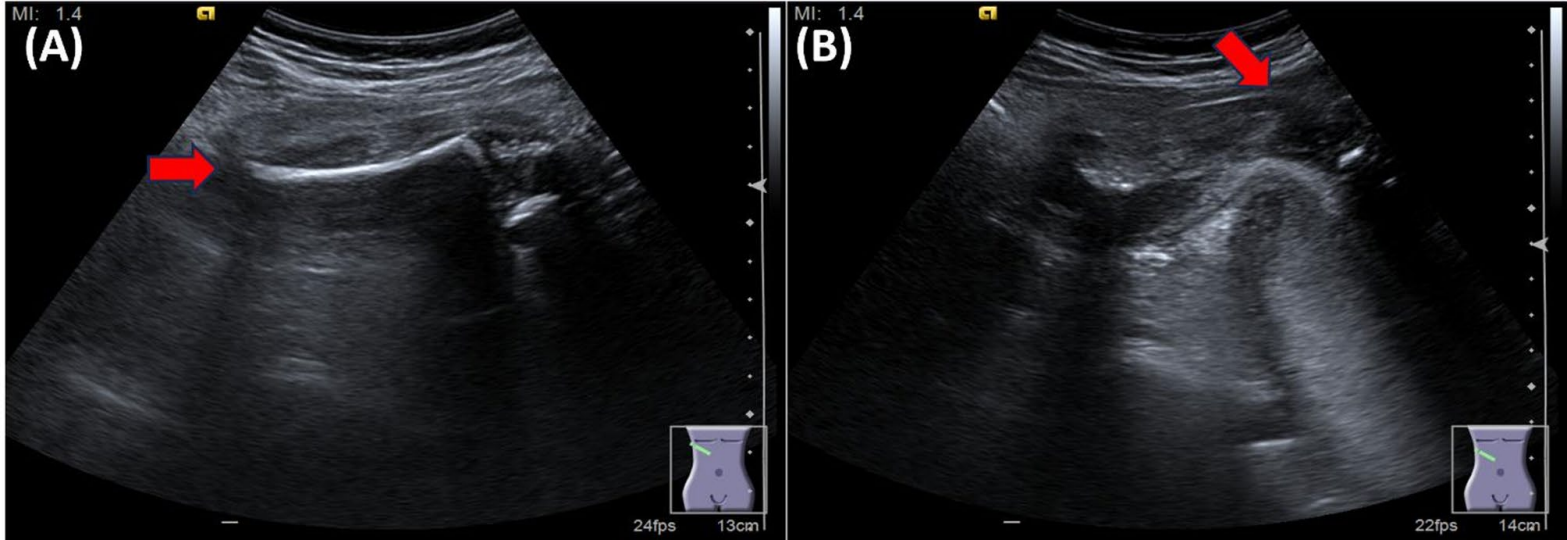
Ultrasonographic findings suggestive of uterine rupture. A Transabdominal ultrasound image of the maternal right upper abdomen showing a suspected fetal thigh protruding outside the uterine contour (red arrow). B Transabdominal ultrasound image of the right uterine myometrium showing suspected fetal thigh protrusion through a uterine defect (red arrow indicates the area of myometrial discontinuity)
